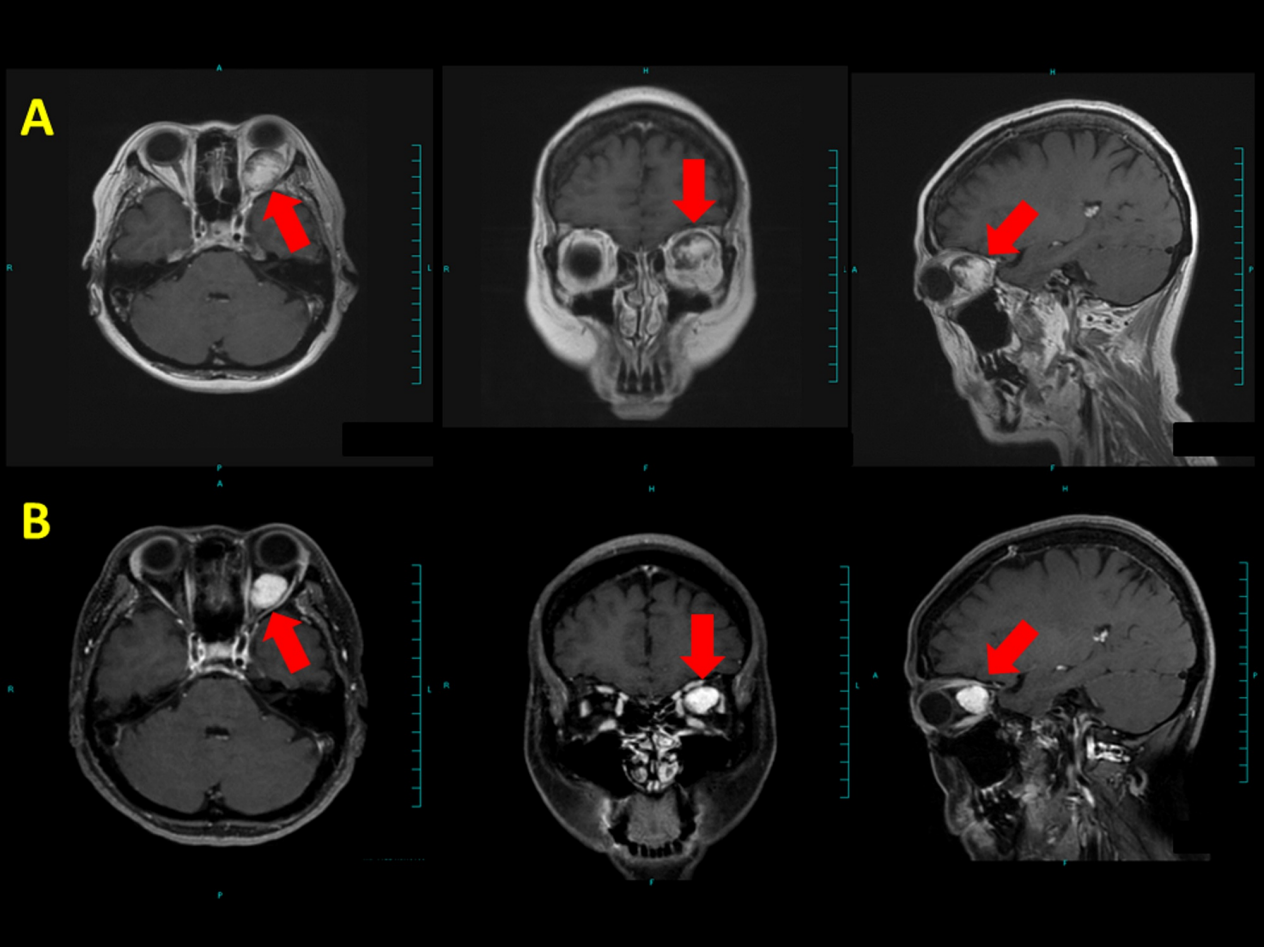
Brain MRI of case 4 Case 4 had extremely decreased visual acuity and exophthalmos. (A) Before treatment and (B) 18 months after treatment. Red arrows denote tumor. The tumor decreased in size; visual acuity and exophthalmos were recovered.
